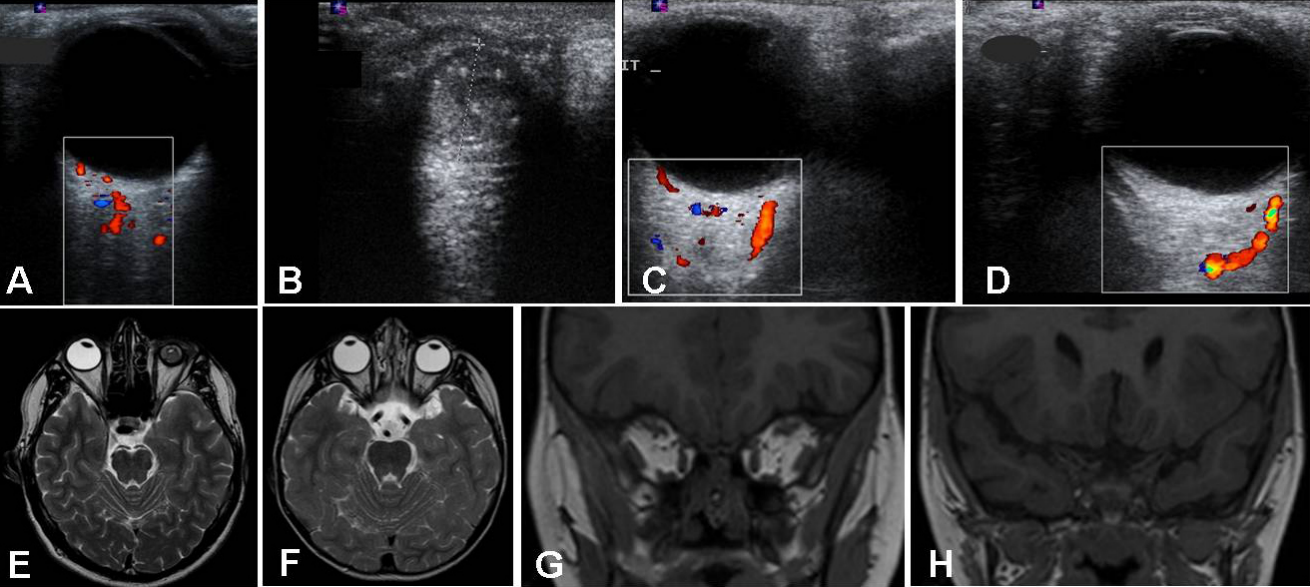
Ultrasound and MRI findings in III:1 and II:1. The father’s (II:1) Doppler ultrasonographic examination. This demonstrates A: a normal right eye with the optic nerve and *arteria centralis retinae*; B: a left microphthalmic heterogeneous eye without the optic nerve visible. C, D: The son’s (III:1) Doppler ultrasonographic examination demonstrating the absence of both optic nerves and corresponding vascularization, but the presence of posterior ciliary vessels (C and D for right and left eye, respectively). The vessels are represented in color. The Doppler examination is represented in the boxes. E: II:1’s axial T2-weighted image in MRI demonstrated a normal right eye and lens and a left microphthalmic eye with thick sclera. F: III:1’s axial T2-weighted image in MRI showed an almost normal morphology of both eyes but the absence of optic nerves (with some remnants of dural sheath), chiasms, and tracts. G-H: Coronal T1-weighted MRI images in the midorbital (G) and intracranial (H) planes. This shows the complete lack of both orbital nerves in the son, III:1.
